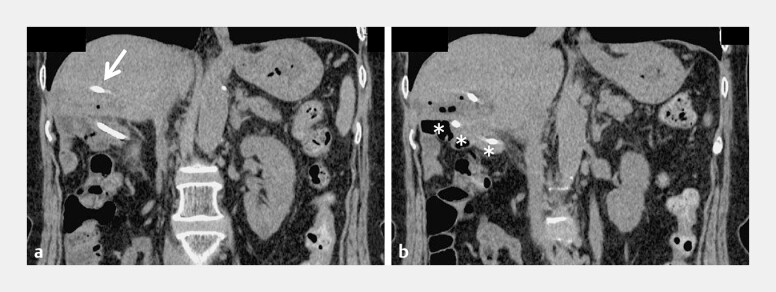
Follow-up abdominal CT performed 1 week after EUS-guided hepaticojejunostomy.
